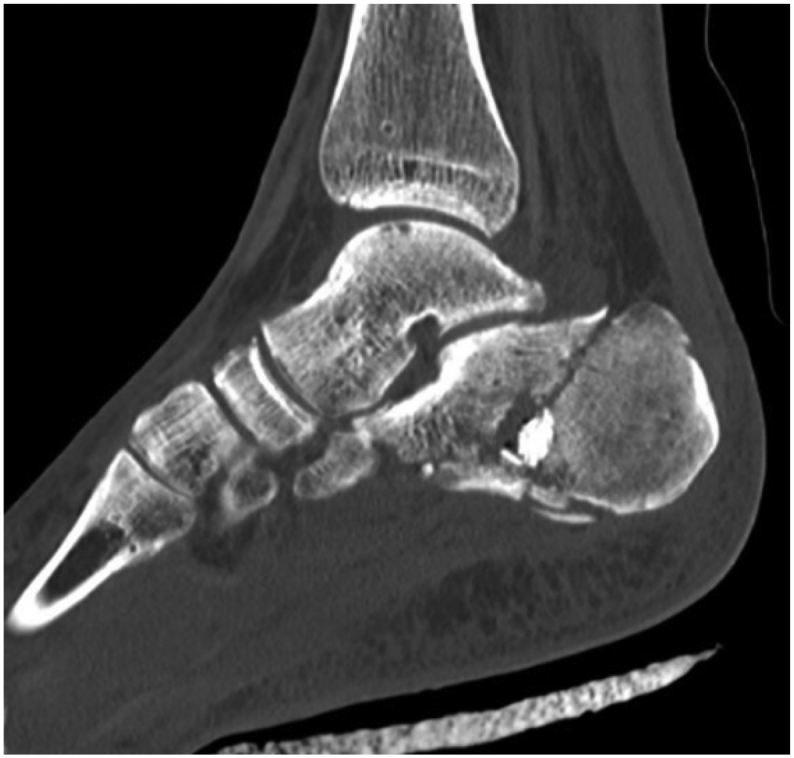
Post-operative sagittal CT slide showing the hyperdense active glass that was used to fill the bone defect.
